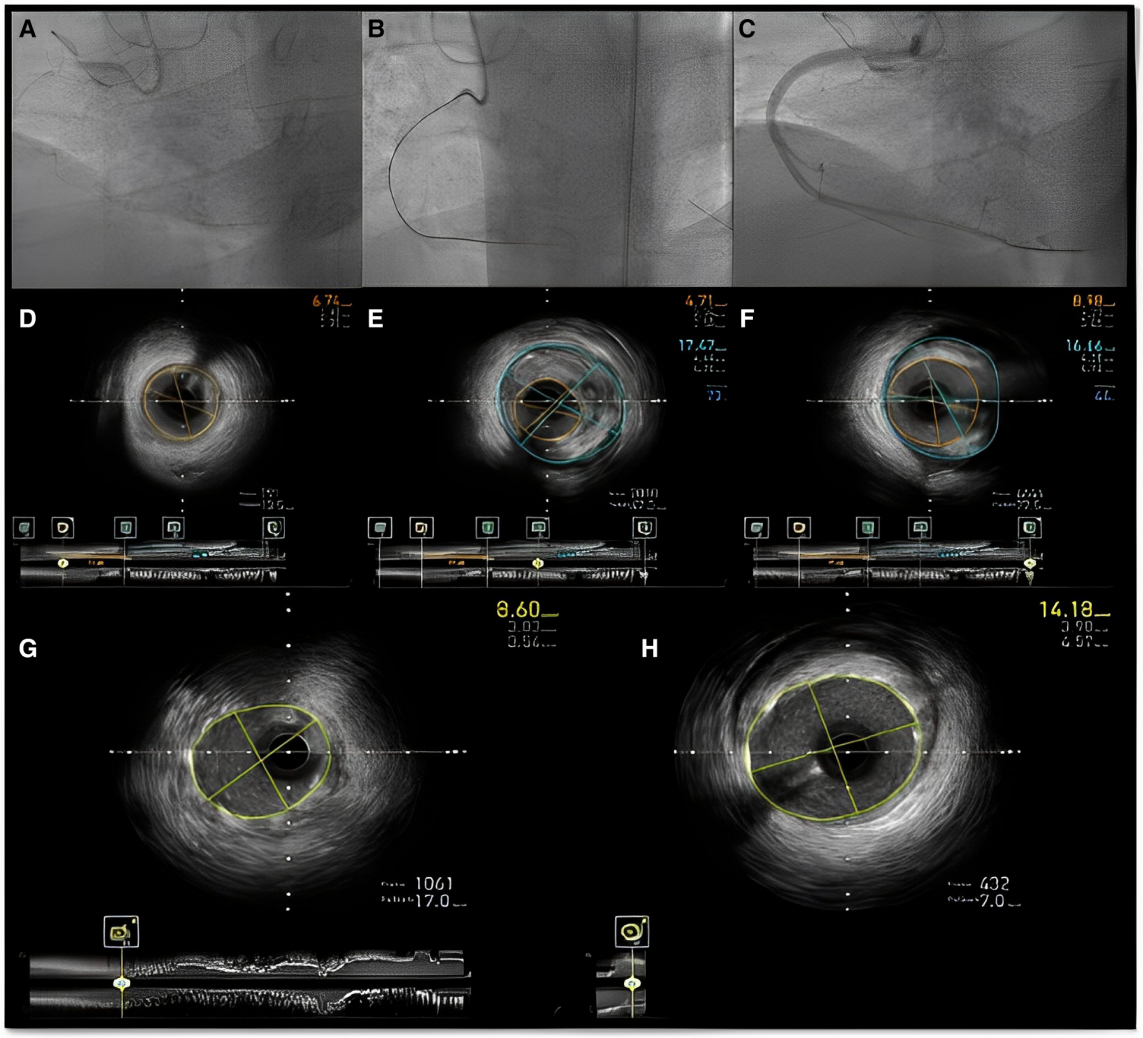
Intravascular imaging (IVUS) guided chronic total occlusion (CTO) percutaneous coronary intervention; (A) initial angiography with contralateral injections to define CTO lesion characteristics; (B) successful antegrade wire crossing; (C) final angiographic result after IVUS guided drug eluting stent (DES) implantation; (D) distal landing zone with reference cross sectional area and diameter estimated at 3 mm; (E) CTO segment cross-sectional area and reference diameter estimated at 4.5 mm; (F) proximal landing zone with reference cross sectional area and diameter estimated at 4.5 mm. The overall lesion length requiring stent coverage from distal landing zone was estimated at 65 mm and therefore two overlapping DES 3.0 × 38 mm and 4.0 × 38 mm were successfully implanted and post-dilated with 3.5 and 4.5 mm non-compliant balloons based on media to media reference diameters as per IVUS measurements; (G) distal stent cross-sectional area with excellent absolute (>5.5 mm^2^) and relative expansion (>100%); (H) proximal stent cross-sectional area with excellent relative expansion (>100%). Used with permission by Elsevier. Kalogeropoulos AS, Alsanjari, O, Davies, JR. et al. Impact of Intravascular Ultrasound on Chronic Total Occlusion Percutaneous Revascularization. Cardiovasc Revasc Med. 2021; 33:32–40.
